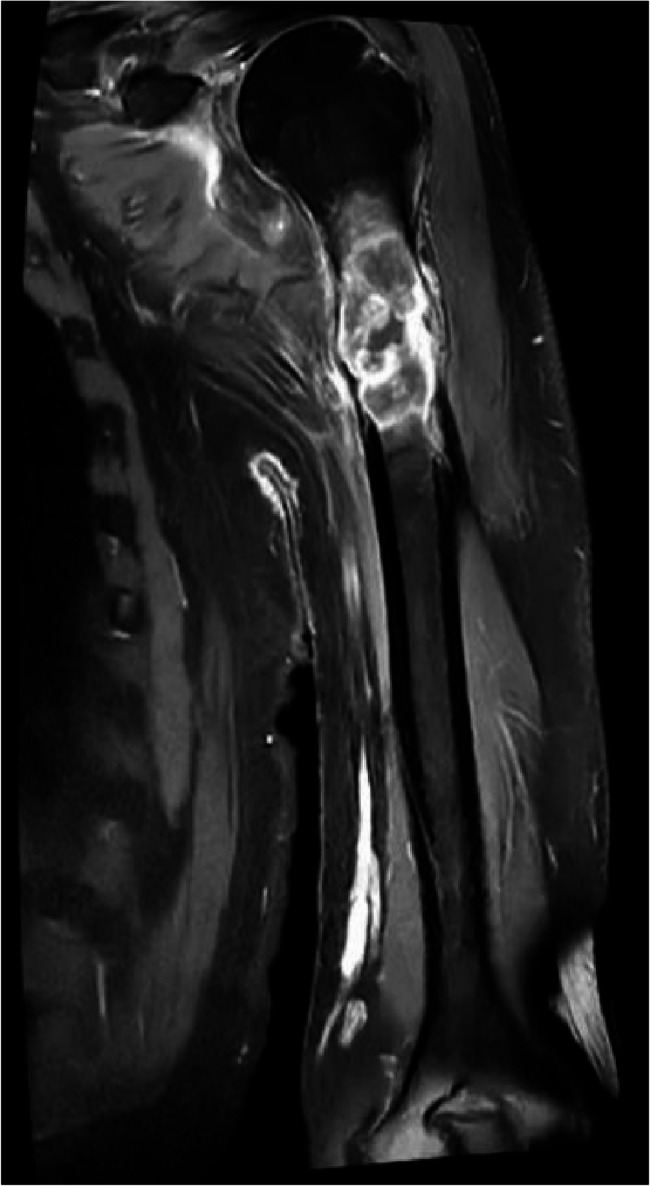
Two months after treatment, follow-up MRI shows: The lesion within the upper medullary cavity of the left humerus has decreased in size compared to before
